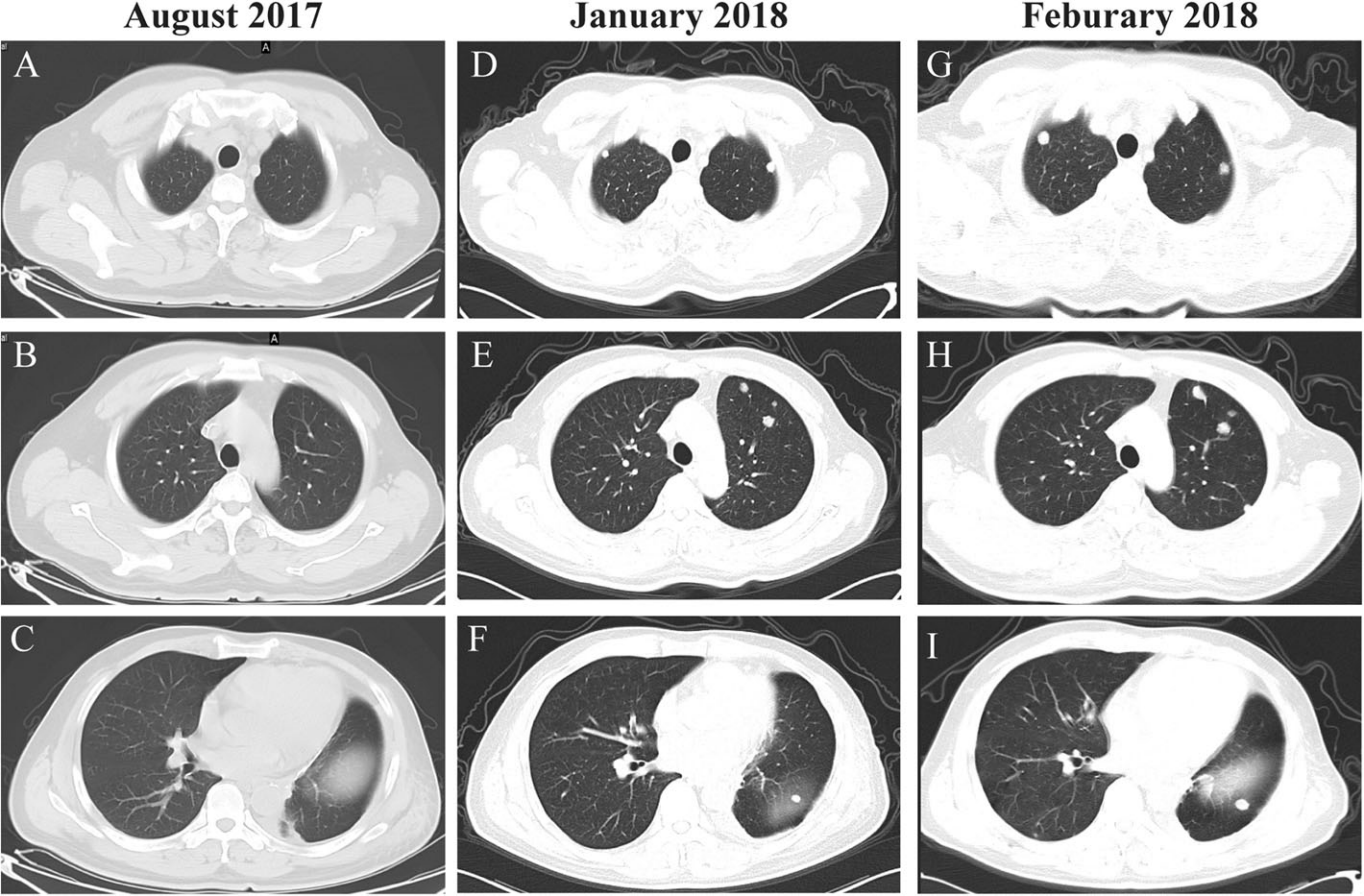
CT scans of the patient. a, b, c CT scan after four cycles of adjuvant chemotherapy with paclitaxel and carboplatin in August 2017. None metastases were observed in the lung or mediastinal lymph node. d, e, f CT scan 5 months after adjuvant chemotherapy, from which many new lung metastases in the entire lung were noted. g, h, i CT scan after two cycles of rh-endostain and docetaxel chemotherapy. The lung metastases became evidently larger
